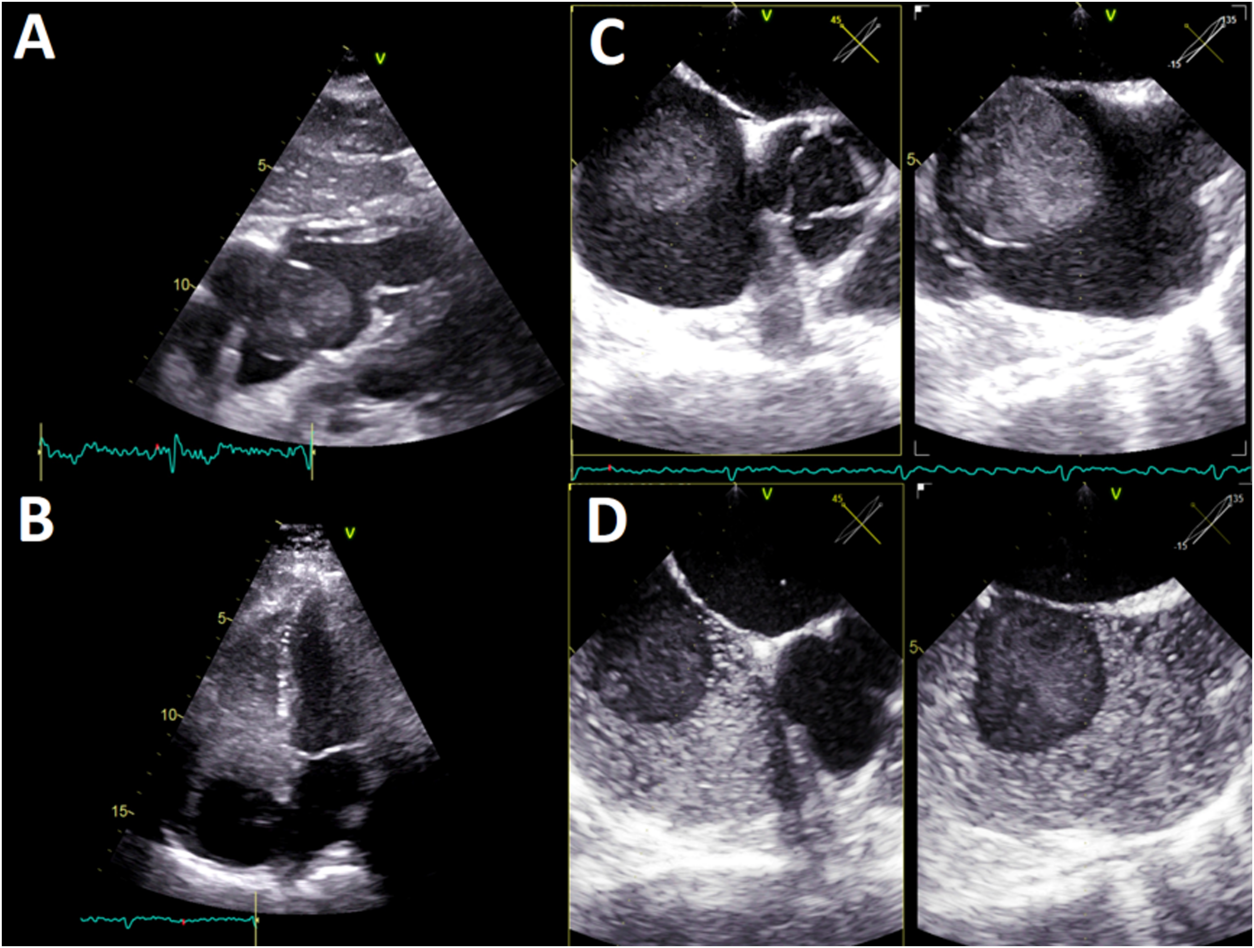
(A) TTE, four-chamber subcostal view. Ovoid mass in the right atrium, with echogenicity almost blood-like and calcification areas. (B) TTE, four-chamber apical view. The agitated saline test shows an anechogenic, 40 × 40 mm^2^, mass in the right atrium implanted in the interatrial septum. No tricuspid valve compromise was observed. (C) TEE, multiplanar image of the interatrial septum (45°; 135°). A homogeneous pedunculated mass with a thin covering was implanted in the middle-low interatrial septum with pendulum movement. (D) TEE, multiplanar image of the interatrial septum (45°; 135°). The agitated saline test depicts anechogenicity of the hematic mass. TTE, transthoracic echocardiography; TEE, transesophageal echocardiography.
